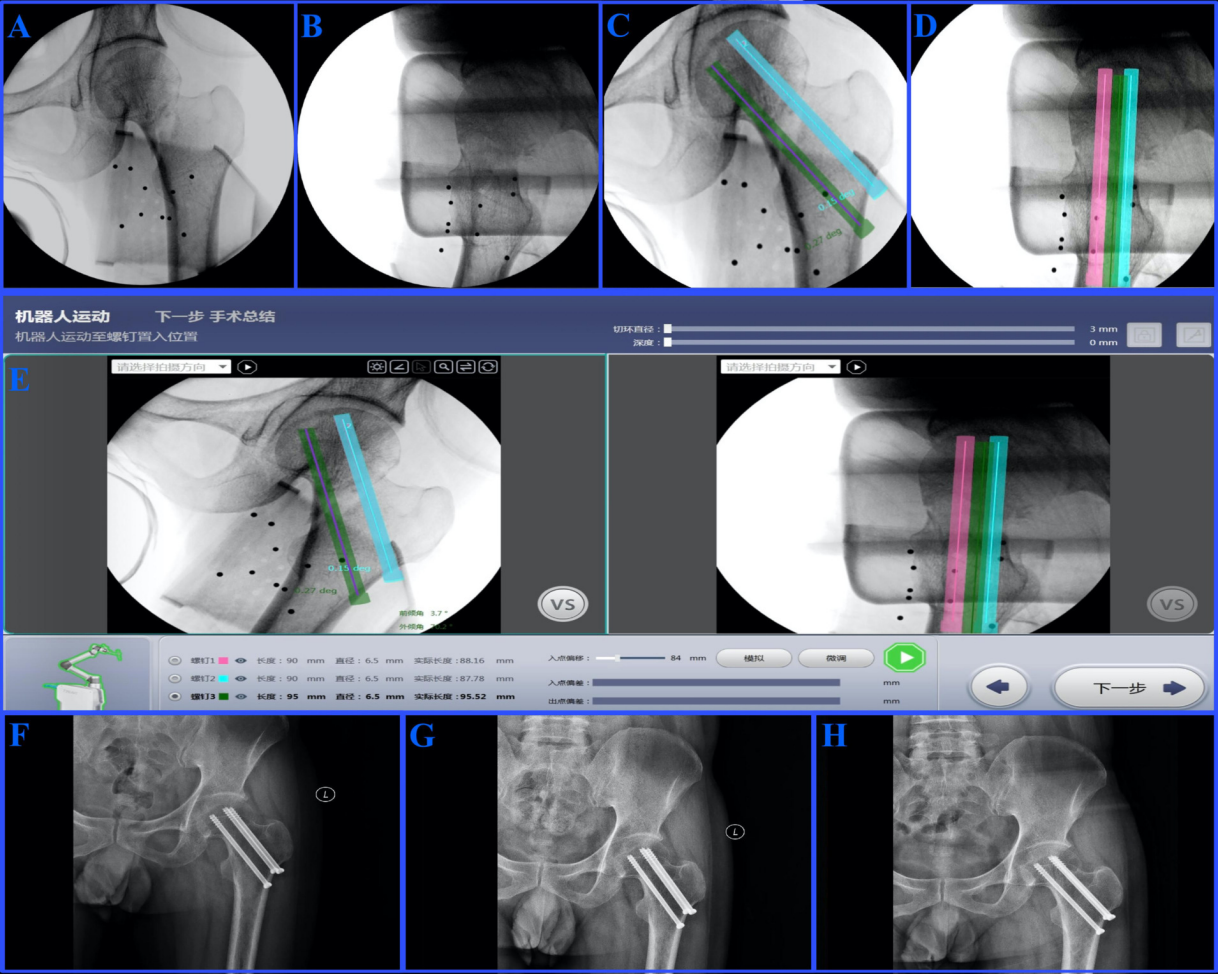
(A,B) Anteroposterior (AP) and lateral x-rays of the fractured femoral neck prior to fixation; (C,D) Planned trajectories for screw insertion displayed on the TiRobot software interface in AP and lateral; (E) AP and lateral views in the software interface showing the calculated screw lengths and alignment for precise fixation; (F) AP x-ray on postoperative day 1 demonstrating proper screw placement and satisfactory fracture reduction; (G) AP x-ray at 12 months postoperative, showing fracture healing and screw positioning; (H) AP x-ray at 20 months postoperative, confirming stable fixation without complications.
